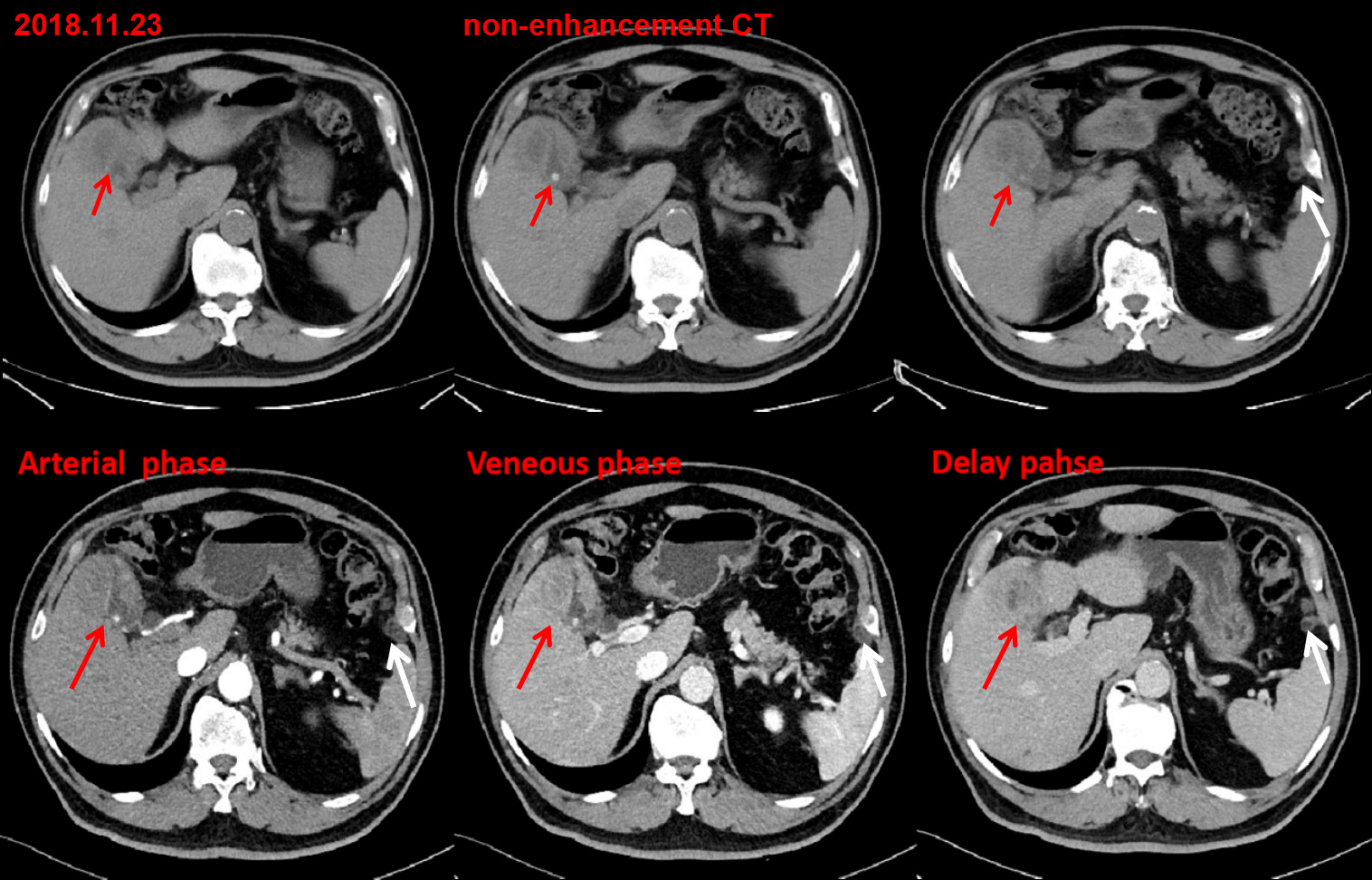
Post-operative 9 month contrast-enhanced CT images detected uneven
